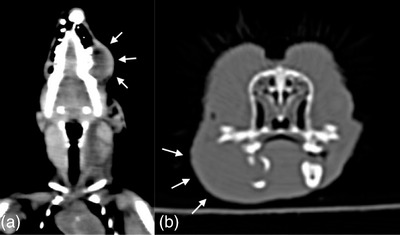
Initial coronal (a) and transverse (b) CT images of the hedgehog. There is a soft tissue swelling that is hypodense relative to the lateral aspect of the left maxilla. The soft tissue swelling is invading the left hemimandible and causing disruption of the underlying bony structure
